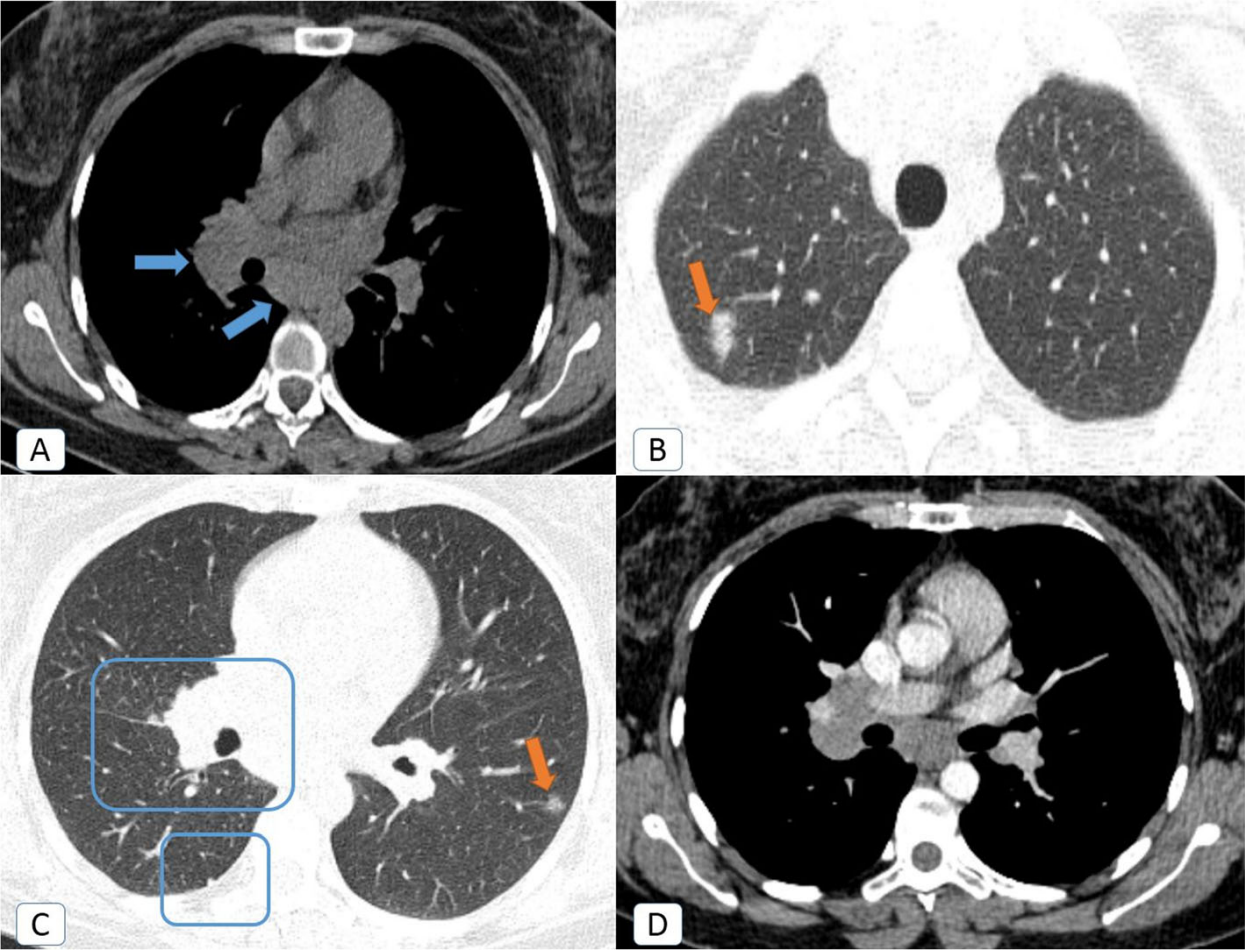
COVID-19 infection on top of incidentally discovered sarcoidosis: a 43-year-old female COVID-19 patient. A Initial axial chest CT (mediastinal window) showing enlarged right hilar and sub-carinal lymph nodes (blue arrows), B lung window CT cut revealed right apical small ground glass patch (orange arrow), C lung window CT at lower level revealed: enlarged right hilar shadow with nearby nodular fissural thickening and lower lobar pleural based nodule (blue squares) as well as left lateral basal sub-pleural small ground glass patch, D follow up contrast-enhanced chest CT after one month and resolution of the ground-glass patches showed persistent enlarged mediastinal and right hilar nodes. Nodal biopsy proved sarcoid disease later on
